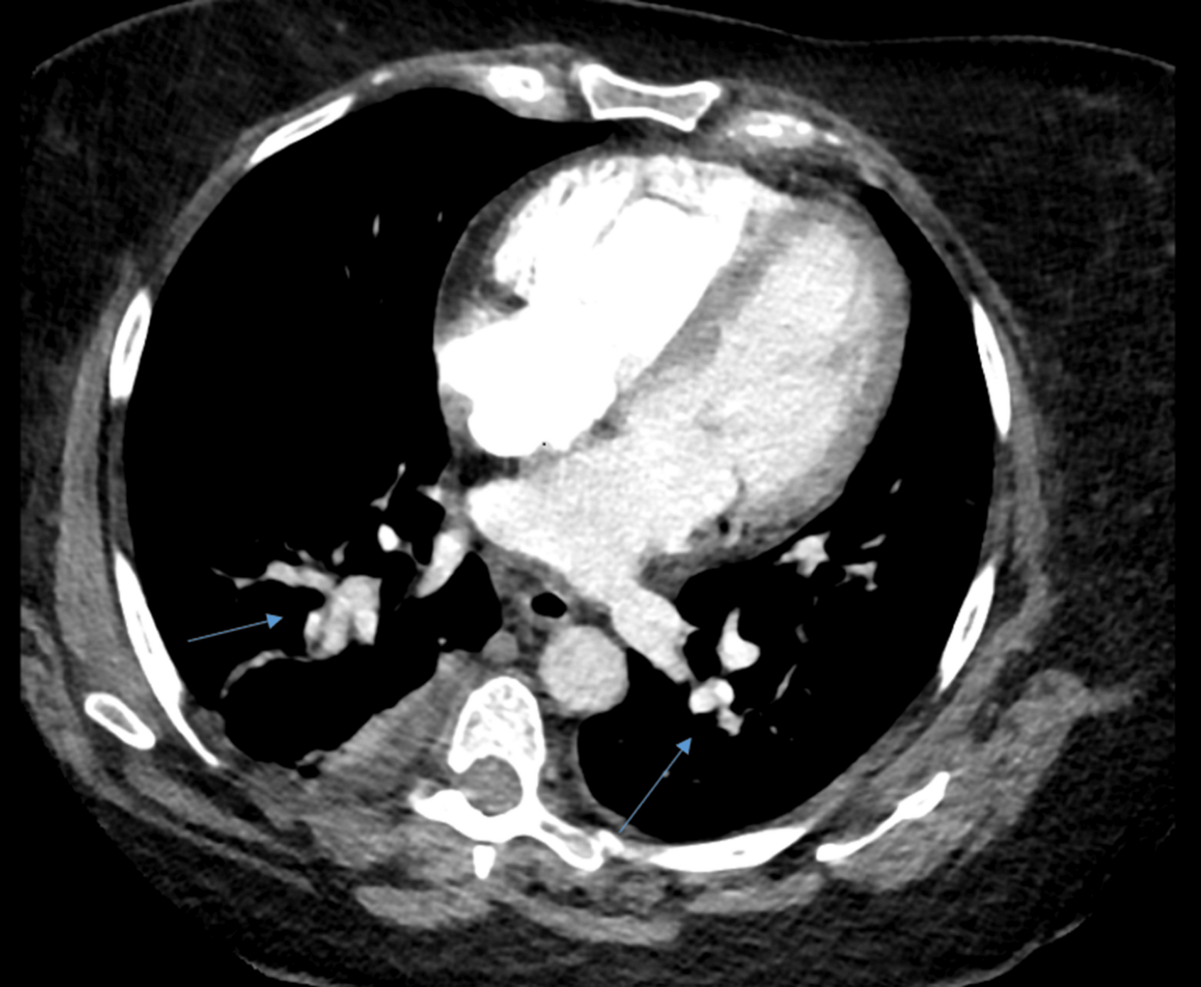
CTPA findings with blue arrows indicating the resolution of pulmonary artery thrombosis after ultrasound-assisted catheter-directed thrombolysis CTPA: computed tomography pulmonary angiography
